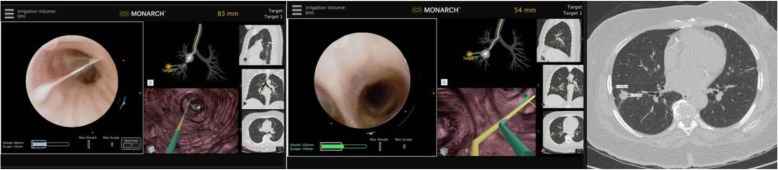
Manually (in yellow) and automatically (in green) generated pathways to a peripheral nodule in RB8 (anterior basal segment of the right lower lobe). The two pathways overlap in the large central airways (left panel) but clearly diverge in 5th generation airway (middle panel). The bronchoscopist must decide which pathway to follow first. In our practice, we follow the manually generated pathway and confirm it with REBUS once at target. If the nodule is not seen on REBUS, the scope is pulled back and the automatically generated pathway is then followed. The right panel shows the nodule biopsied in this case (a 15.8 X 15.7 mm nodule in RB8) REBUS: radial probe ultrasound
